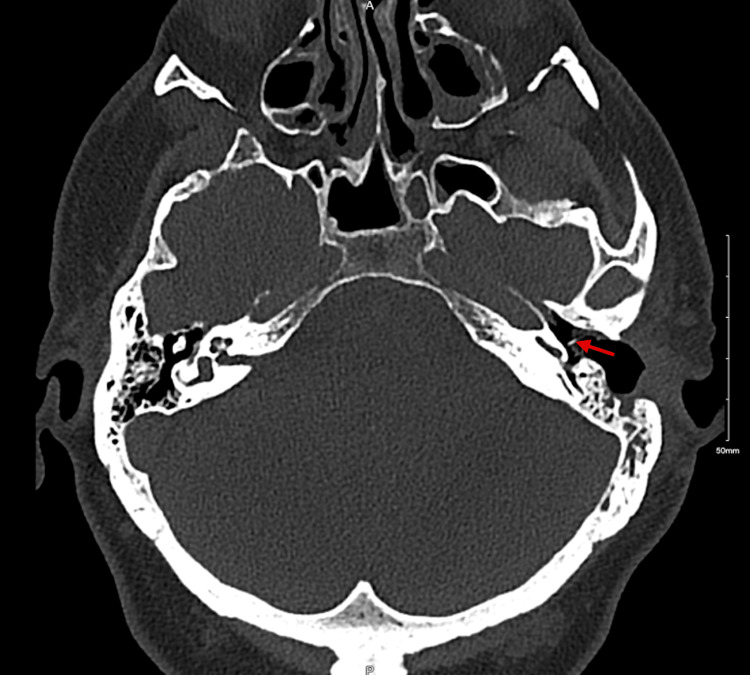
Axial section of CT temporal bone scan Axial slice demonstrating disease recurrence in the left ear with ossicular chain erosion. The right middle ear cavity appears normal.
